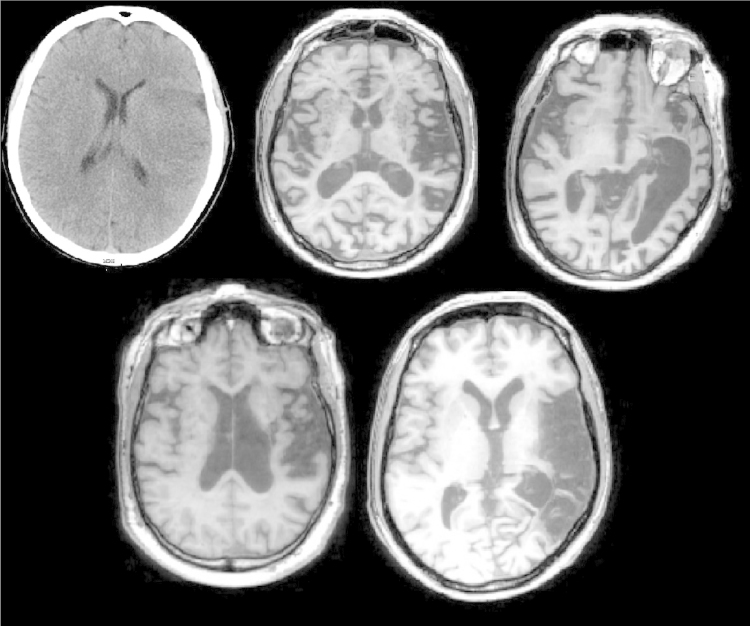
Individual RH lesions CT/MRI scans, showing the slice with the greatest lesion. From top left: ASW, DGX, SYN, NDW, DNQ.
